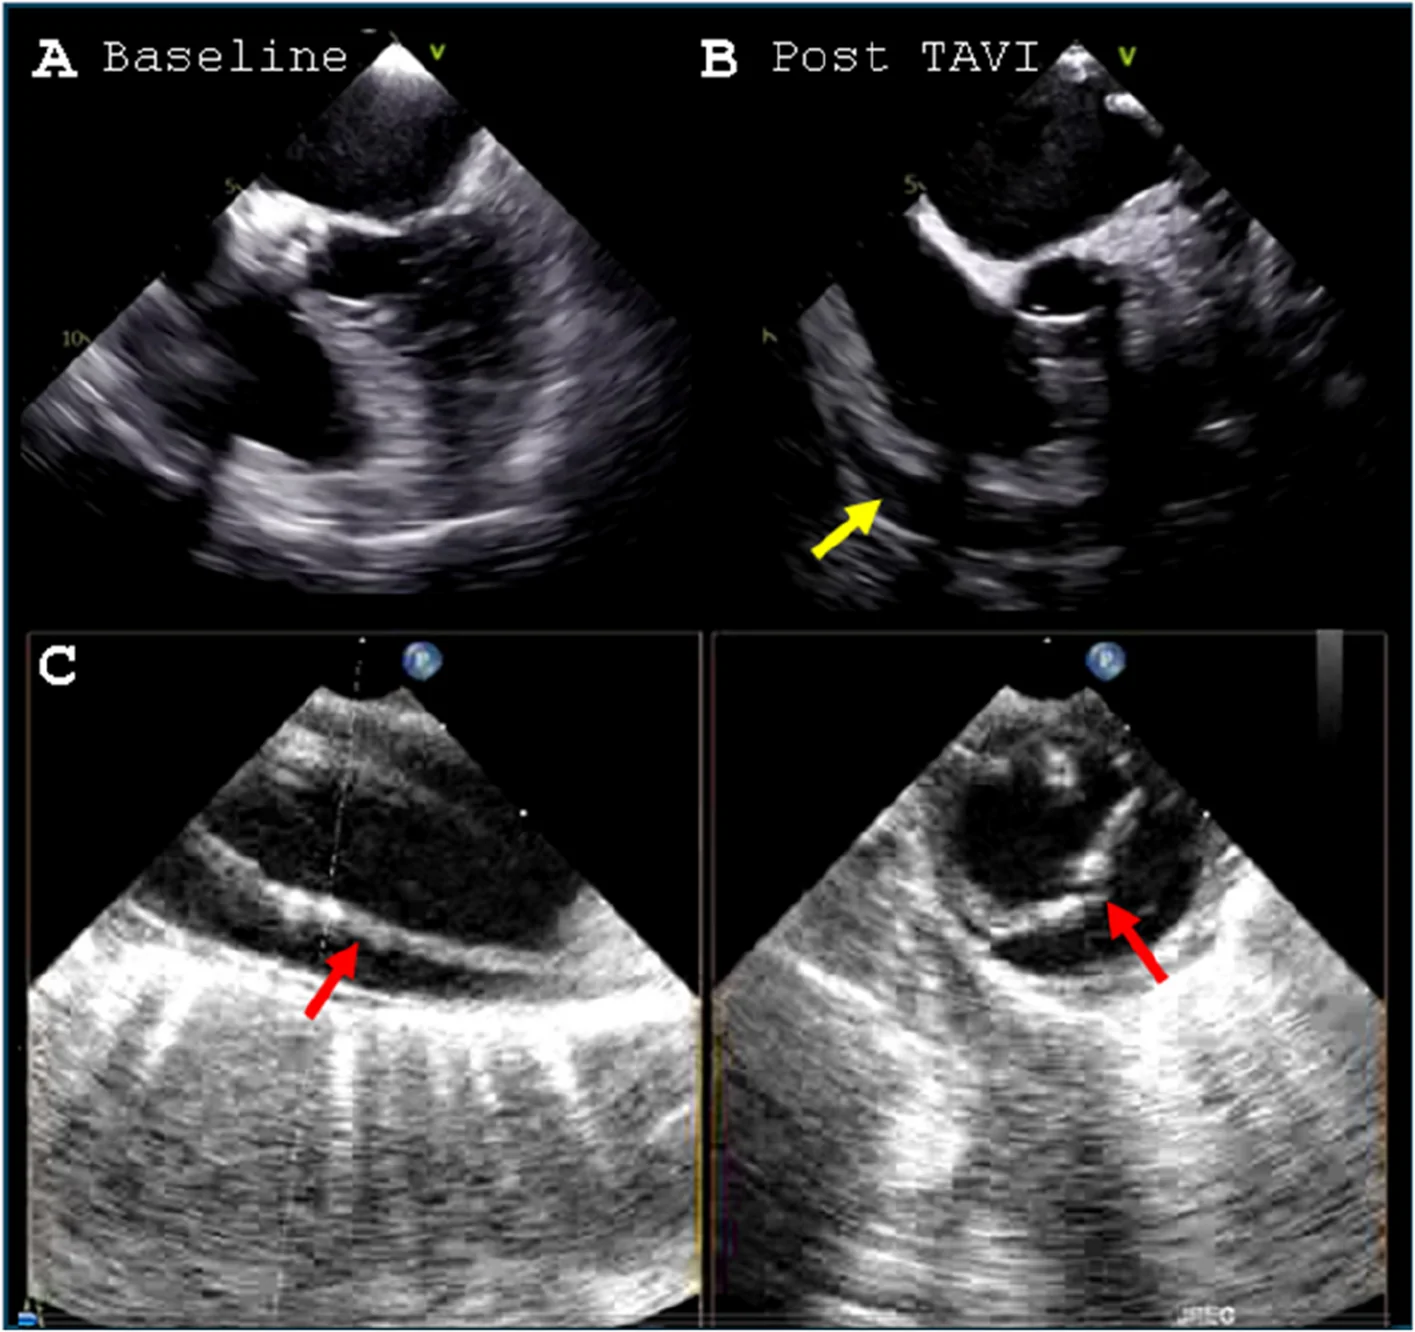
Potentially life-threatening complications detected by intraprocedural transesophageal echocardiography (TEE) during transcatheter aortic valve implantation (TAVI) procedure. A Pre-TAVI TEE at baseline showing minimal pericardial effusion. B Post-TAVI TEE showing a moderate size pericardial effusion (yellow arrow) caused by right ventricular perforation from pacing wire, that was promptly identified and timely drained without any hemodynamic compromise. C Aortic dissection demonstrated on biplane TEE imaging in a patient who developed hypotension. Dissection flap was visualized in the descending aorta (red arrow)
